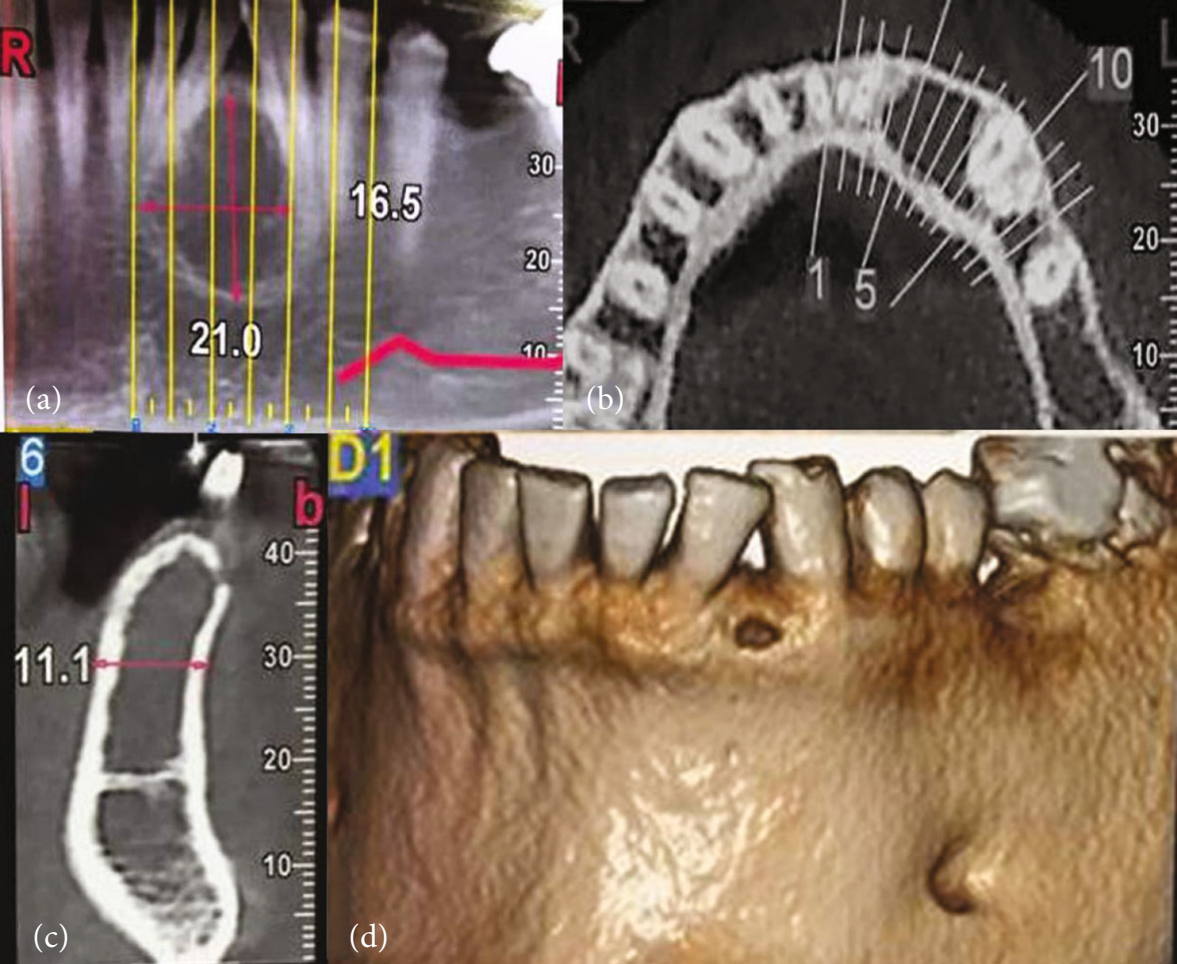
Cone beam computed tomography assessment of the lesion: (a) a well-defined radiolucent lesion with root separation in panoramic view; (b) mesiodistal extension of the lesion in axial view; (c) cross-sectional view; (d) 3D assessment.
